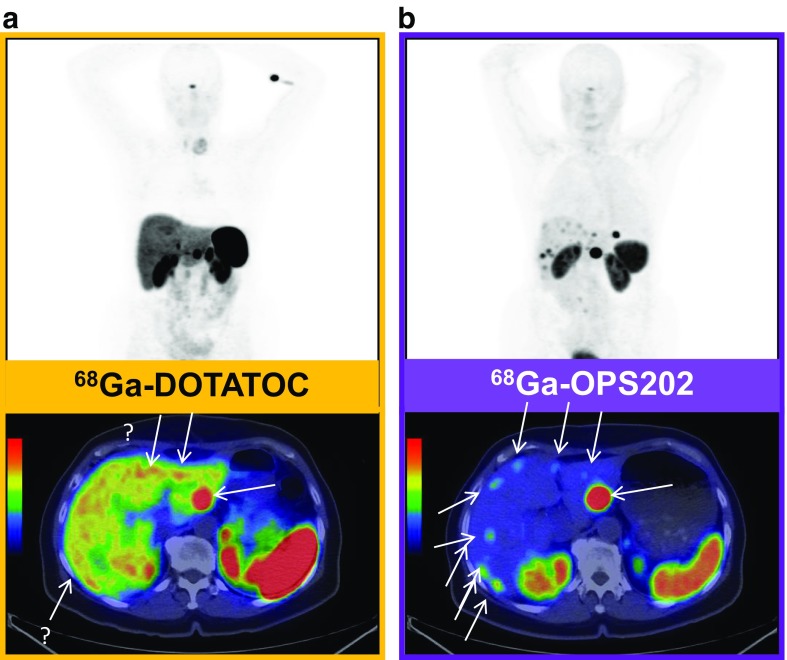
SSTR2 antagonist ^68^Ga-OPS202 for somatostatin receptor PET/CT in gastroenteropancreatic NET. ^68^Ga-OPS202 shows higher sensitivity and image contrast (b) than the ^68^Ga-DOTATOC (a)
